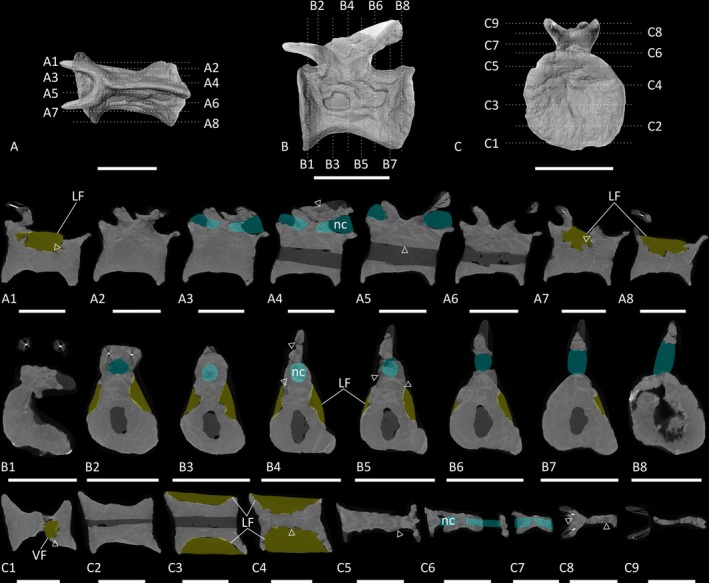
CT scan of *Giraffatitan brancai* caudal vertebra 24 MB.R.5000.23. (A–C) 3D rendering (A) dorsal view, (B) left lateral view, (C) anterior view; (A1–A8) CT scan slices in parasagittal cross‐section, (B1–B8) CT scan slices in transverse cross‐section, (C1–C9) CT scan slices in horizontal cross‐section. LF, lateral fossa; nc, neural canal; VF, ventral fossa. Key: Blue highlight, neural canal; yellow highlight, centrum fossa; white arrow, pneumatic bone texture. Scale bars = 100 mm.
